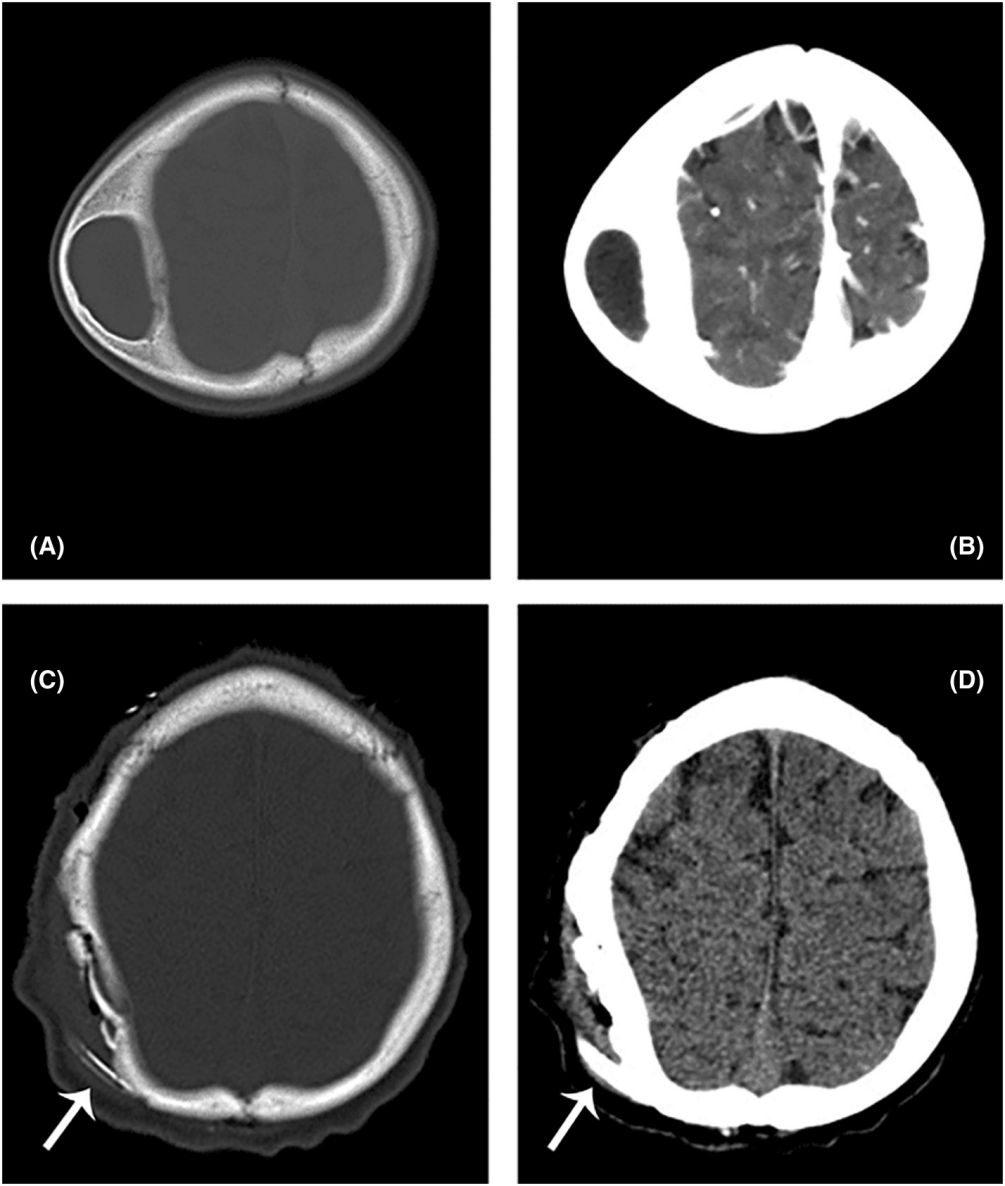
Pre‐ and postoperative images. (A) Axial bone view CT scan of the lesion, (B) axial post‐contrast CT scan of the lesion without any enhancement, (C) Postoperative axial bone and brain (D) view CT scan. Arrows show the drain tube.
